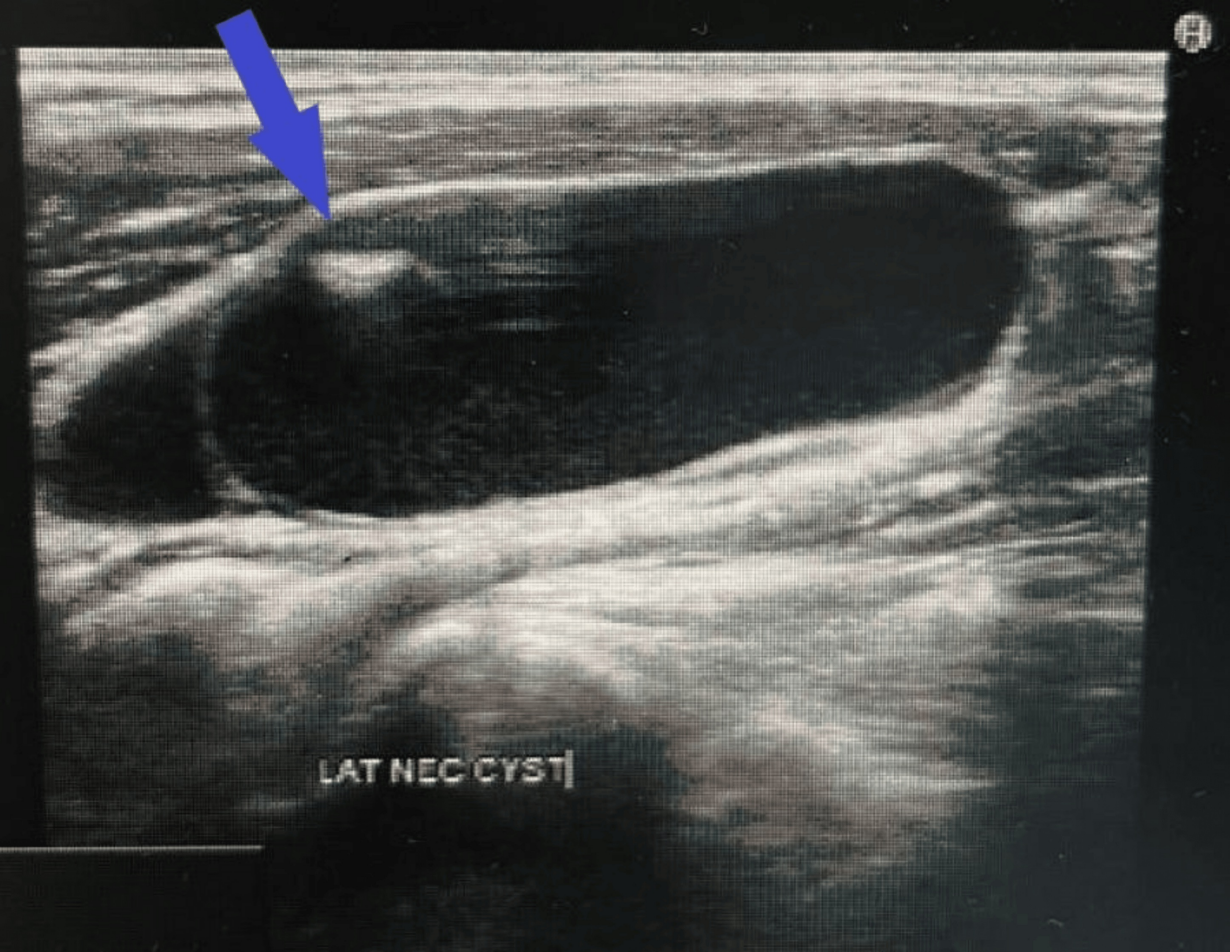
Ultrasound of the neck A 5.2 x 1.9 cm thick-walled lobulated cystic lesion in the left lateral neck, inferior to the left sternocleidomastoid muscle, with slight mass effect on the adjacent left jugular vein. It demonstrates internal solid components (blue arrow), debris, and a few septations.
